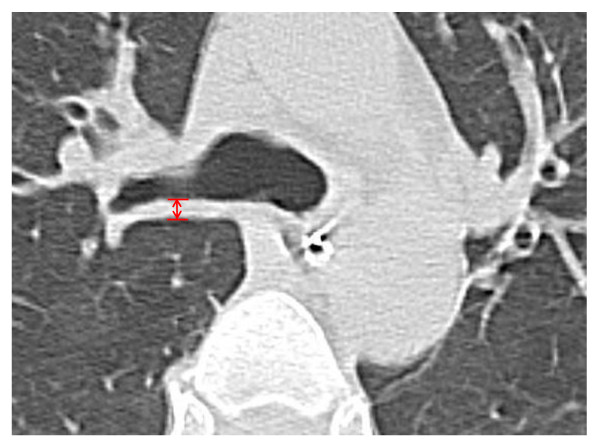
Indices of bronchial wall thickness on HRCT scan. Measurement position of bronchial-wall thickness (arrows). HRCT, helical high-resolution computed tomography.
